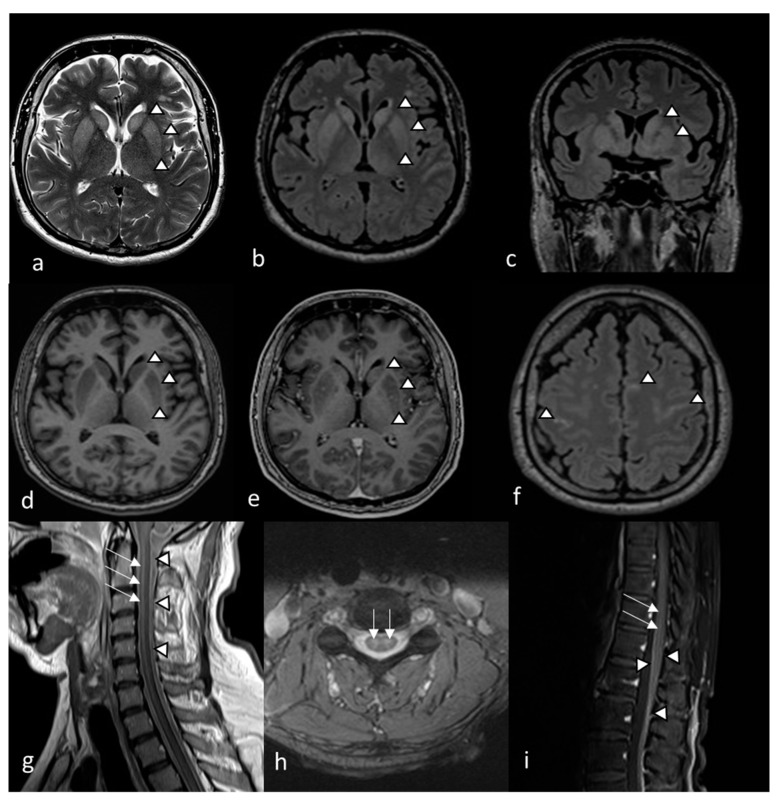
MRI findings on the 14th day of illness in a patient with TBE. The MRI showed symmetric diffuse T2wi (a) and FLAIR (b). Hyperintensity of the basal ganglia, especially the putamen and caudate nucleus, with a slightly less pronounced increase in the signal of both thalami (arrowheads) on axial (a,b) and coronal (c) images, consistent with edema. Bilaterally decreased T1wi signal in the putamen and caudate nucleus (d) followed by patchy contrast enhancement (e) on axial images (arrowheads), implying basal ganglia inflammatory lesions. Meningeal contrast enhancement on FLAIR (f) and leptomeningeal contrast enhancement in the cervical medulla (g) and medullary cone (i), correlating with meningeal/leptomeningeal inflammation (arrowheads). Symmetric T2wi signal increase found in cervical medulla (h) with contrast enhanced areas delineated in cervical (g). Lumbar spinal cord (i) correlating with myelitis (arrows).
